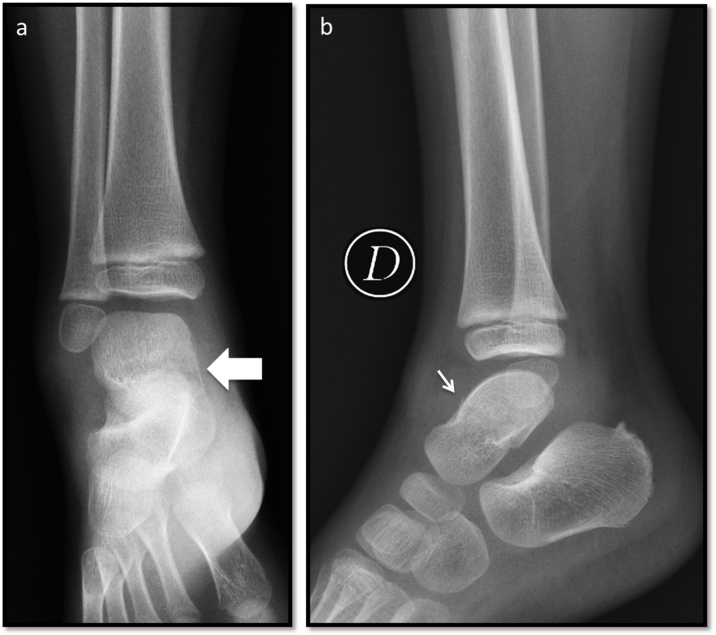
a. Anteroposterior x-ray of the ankle shows a lineal, horizontal, radiolucent image of the talus body (arrow). b. Lateral x-ray of the ankle, the radiolucent trace is less evident, shows an irregularity in the cortical bone (thin arrow).
